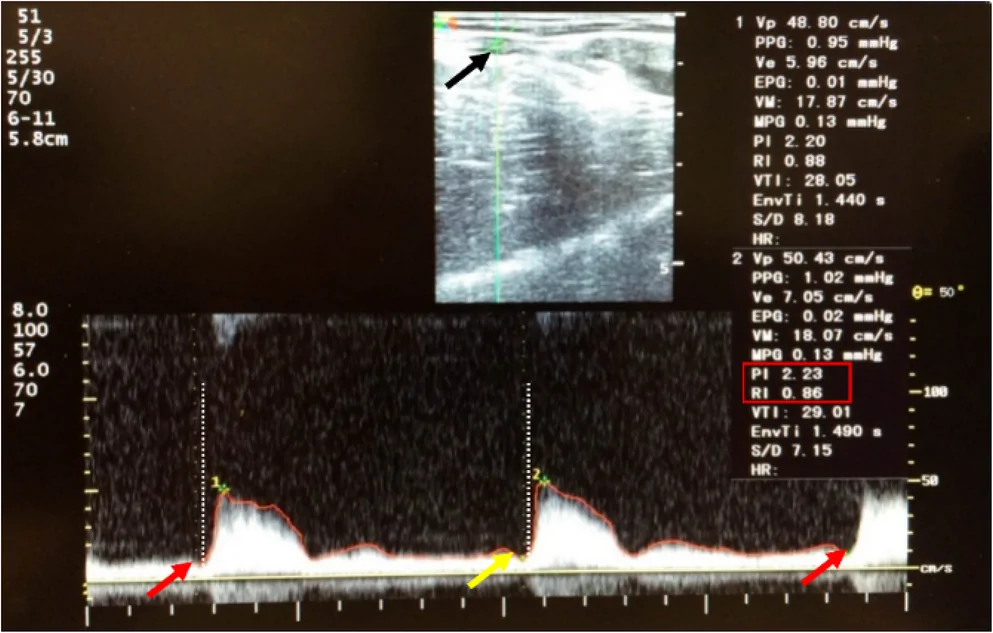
Spectral Doppler ultrasonography of the dorsal branches of the uterine arteries for evaluation of the resistance index (RI) and pulsatility index (PI). The red box identifies the Doppler sample volume used to generate the spectral waveform for calculation of RI and PI. The black arrow indicates the position of the ultrasound cursor on the uterine artery. The onset of systole and the end of diastole are indicated by the red and yellow arrows, respectively. Source: personal archive
